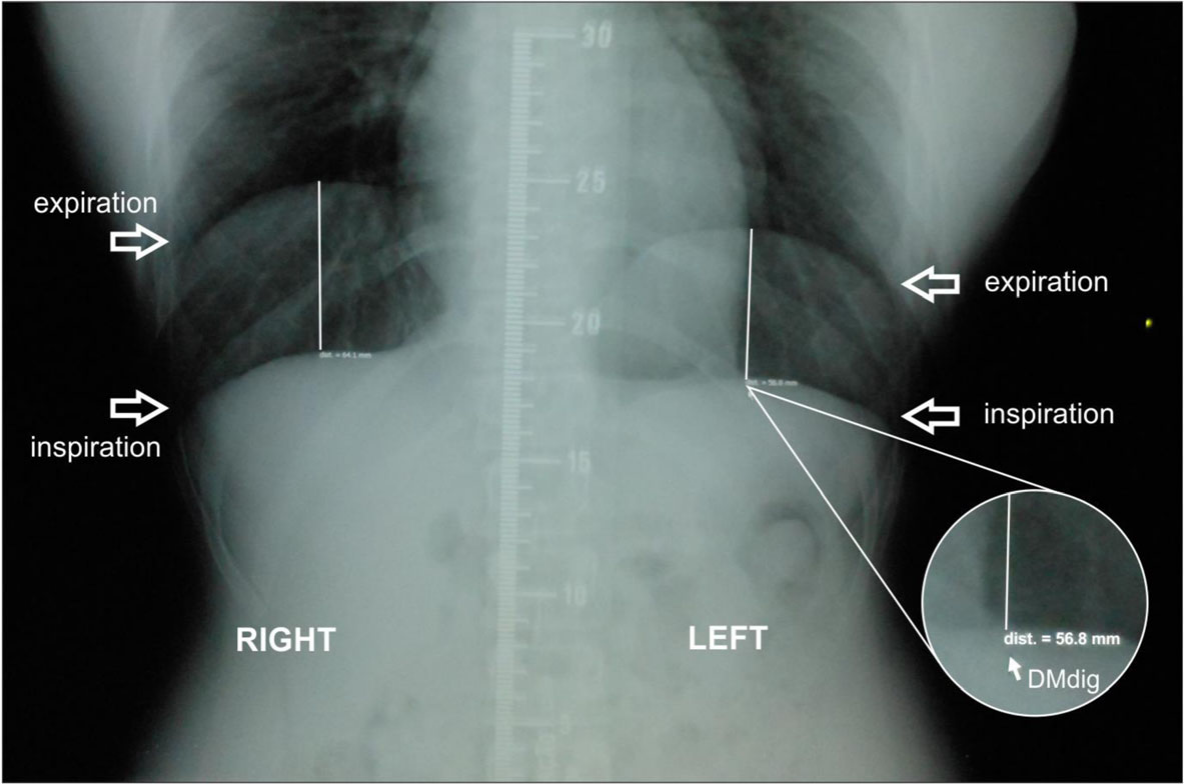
Measurement of diaphragm mobility obtained by the software. Digital radiography of the chest in anteroposterior view (AP) during maximal expiration and maximal inspiration conducted on the same film. Measurements of the mobility of *right* and *left* hemidiaphragms were obtained by the software of the device using the ruler on the image for calibration. Source: author's own production
